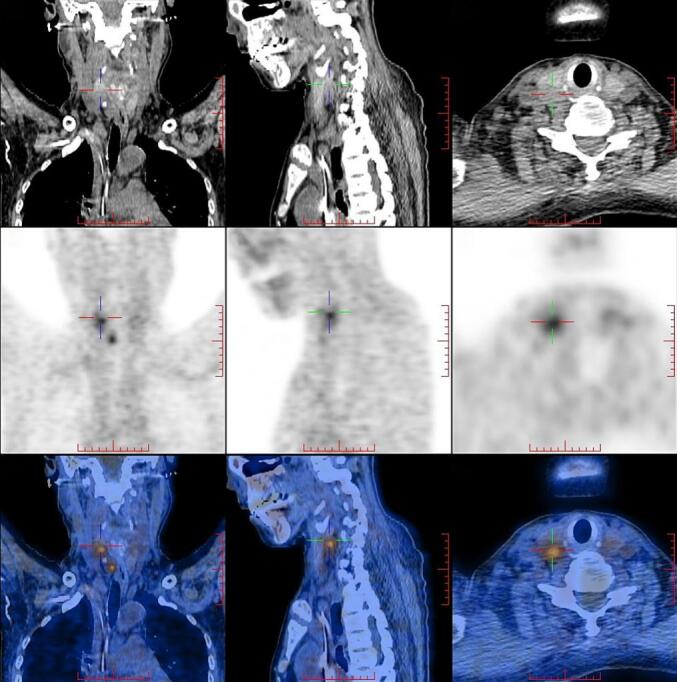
The PET/CT scan of Case 6 shows several slightly hypodense nodules in the parathyroid region, with the largest located on the right side, measuring approximately 16 × 11 mm. These nodules exhibit radiotracer uptake, with an SUVmax of approximately 4.3.
